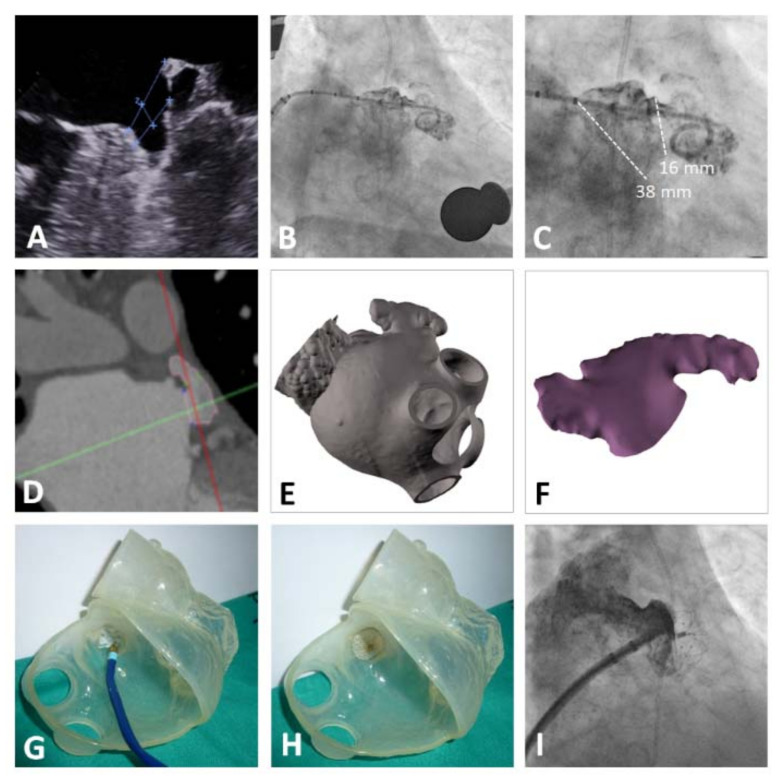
Small, slight, and multilobar LAAC. Legend: (A) TEE image of the LAA. (B,C) LAA angiography. (D) CT of the LAA. (E,F) Three-dimensional reconstruction of the LAA. (G,H) 3D-printed model to help with selection of the device size and plan the procedure. (I) Successful implantation of a 16-mm LAmbre™ device.
